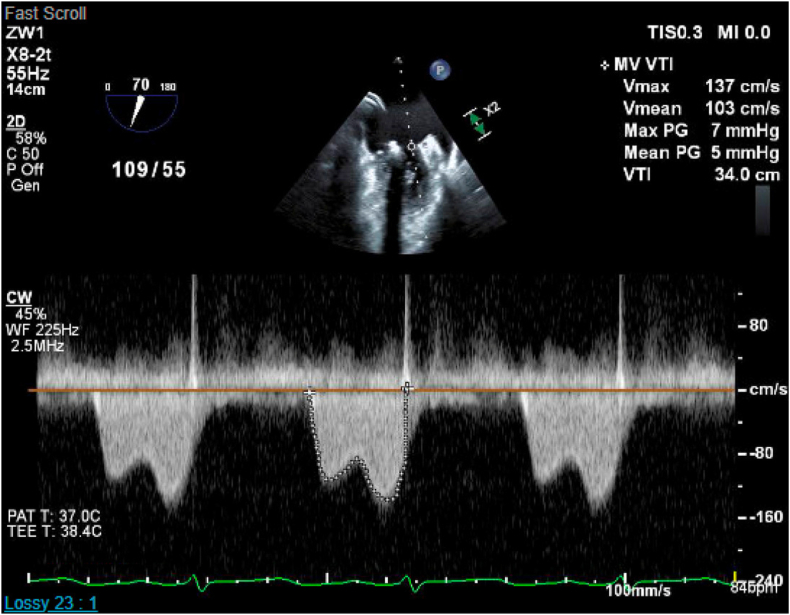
Intraprocedural TEE showing mitral mean gradient of 5 mmHg by CW Doppler before implant release. Abbreviations: CW, continuous wave; MI, magnification; MV, mitral vave; PAT, patient; PG, peak gradient; TEE, transesophageal echocardiography; VTI, velocity time integral.
